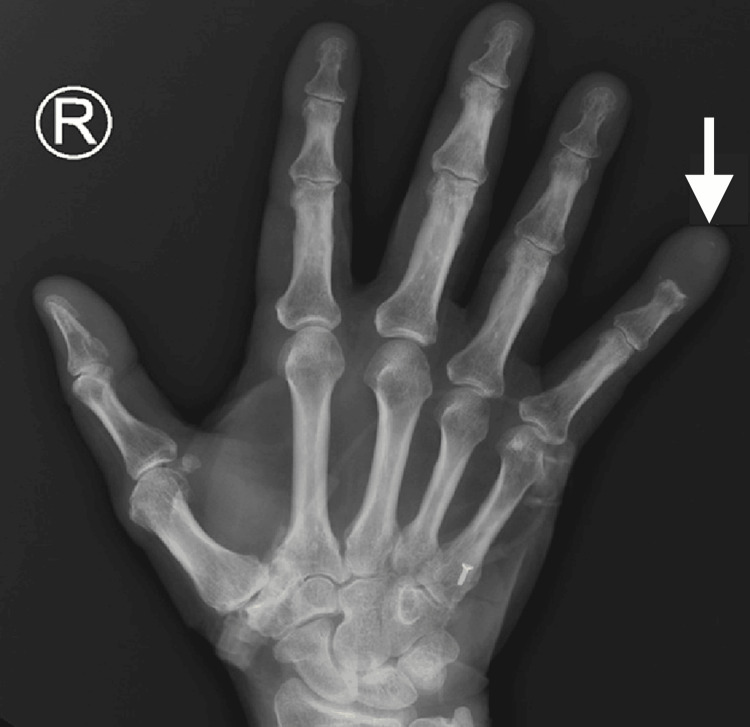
Diagnostic anteroposterior (AP) X-ray imaging of the right hand. Destructive lesion of the fifth distal phalanx (arrow).
